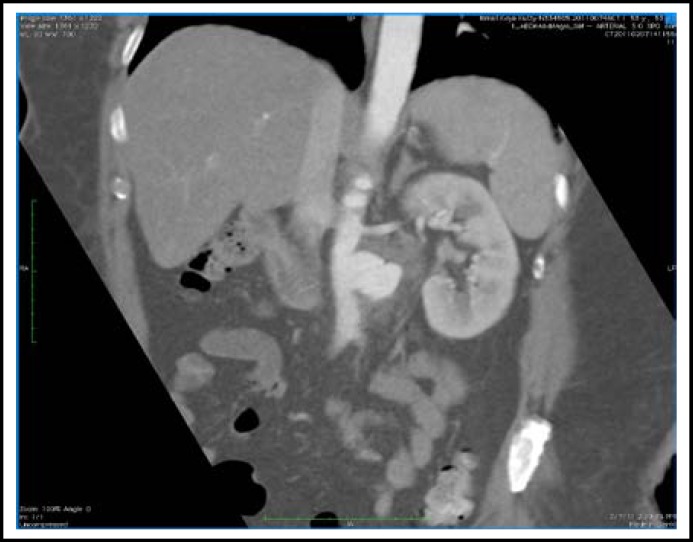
Coronal CT section of the patient’s abdomen showing a left saccular infrarenal aortic aneurysm
